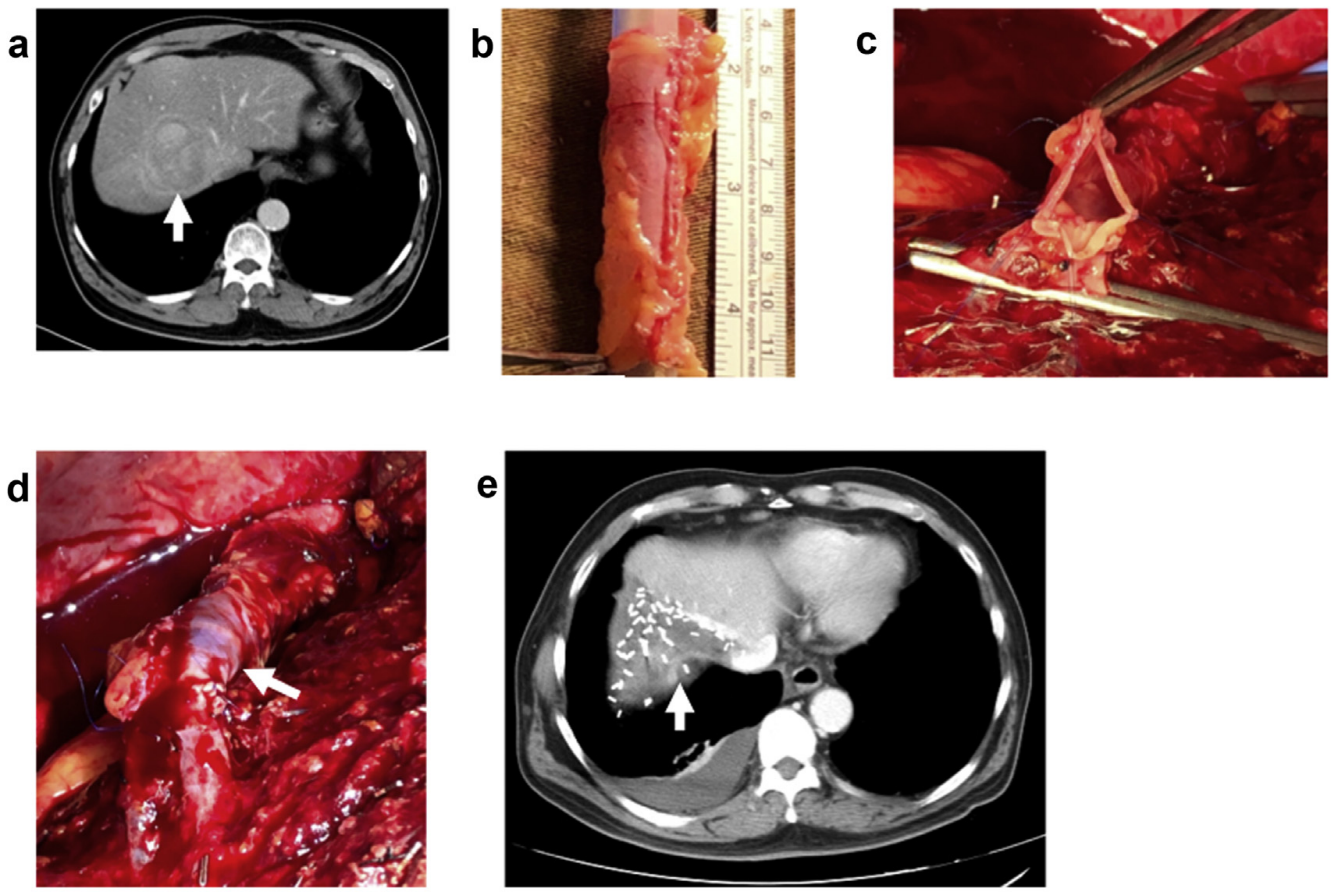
a, A 6-cm hepatocellular carcinoma (*arrow*) at segment VII/VIII of the liver. b, Fashioning the falciform ligament tube graft. c and d, Anastomoses of the interposition tube graft (*arrow*) to the right hepatic vein (HV). e, Postoperative computed tomography scan showing patent falciform ligament tube graft (*arrow*).
